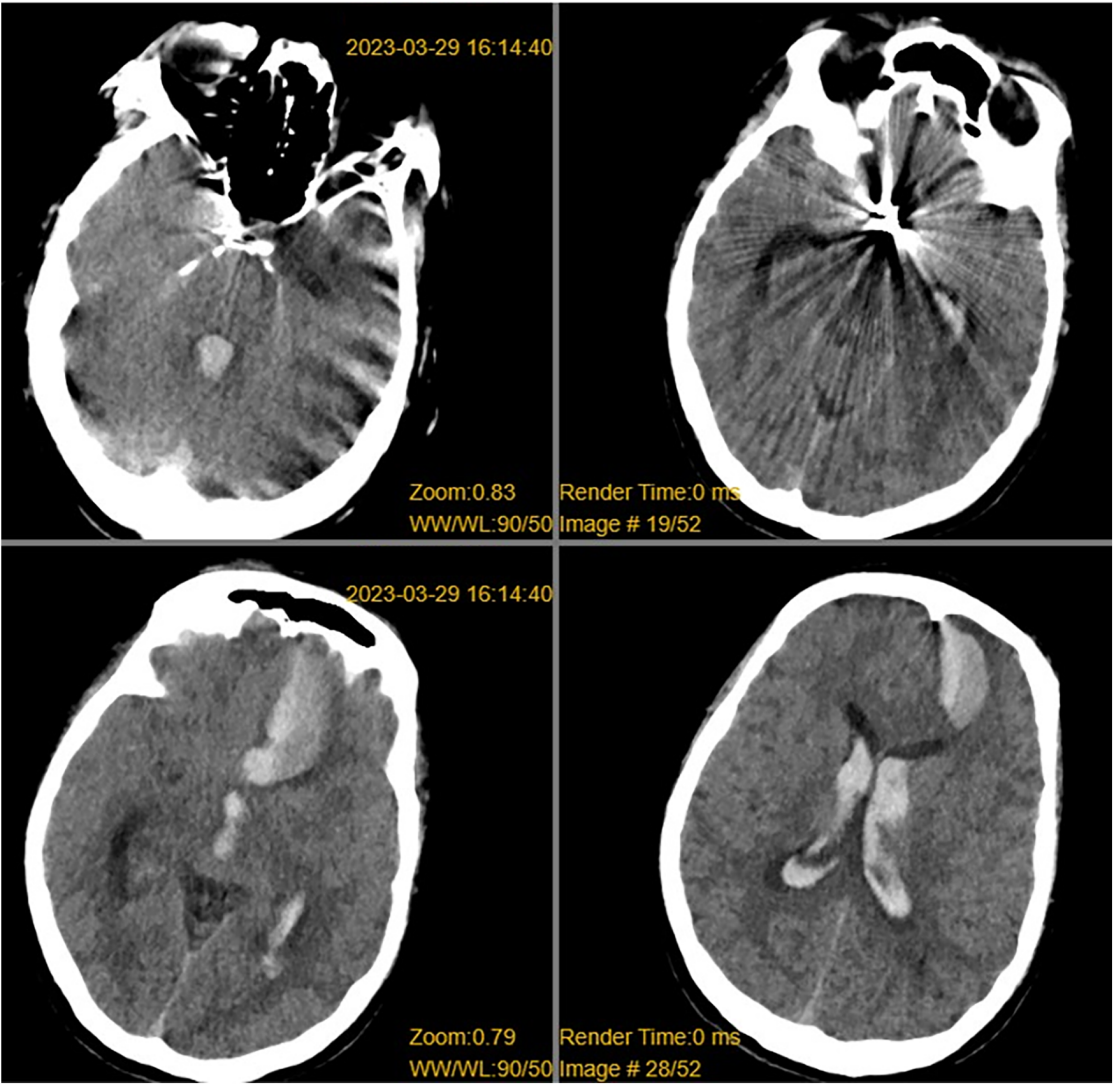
CT revealed a left frontal lobe hematoma, intraventricular hemorrhage, subarachnoid hemorrhage, and metallic artifacts in the sellar region.
